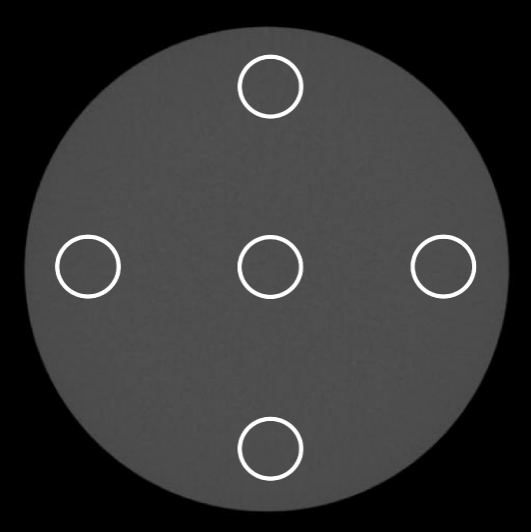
The circular regions of interest (2.0 cm diameter) for measurement of the computed tomography (CT) value and noise level.
